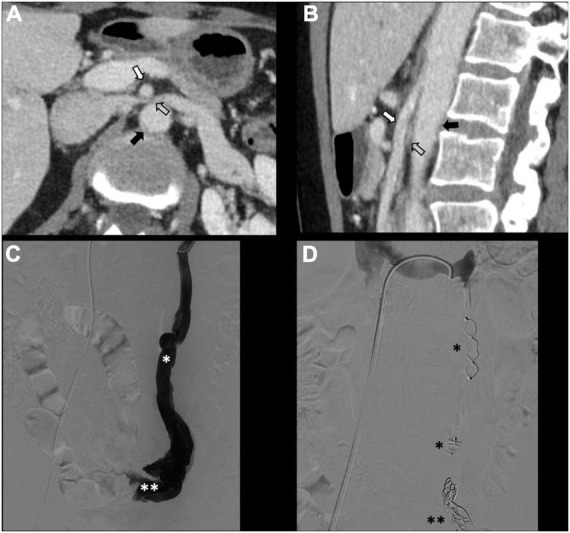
Type IIb pelvic varices in a 54 yo female due to nutcracker syndrome. (A,B) Computed tomography (CT) phlebography shows the left renal vein (open arrow) compressed by the mesenteric artery (white arrow) and aorta (black arrow). (C,D) Conventional phlebography shows dilated left ovarian vein (single white asterisk) with left-sided pelvic varices (double white asterisks), treated with multiple plugs (single black asterisk) and coils (double black asterisks).
